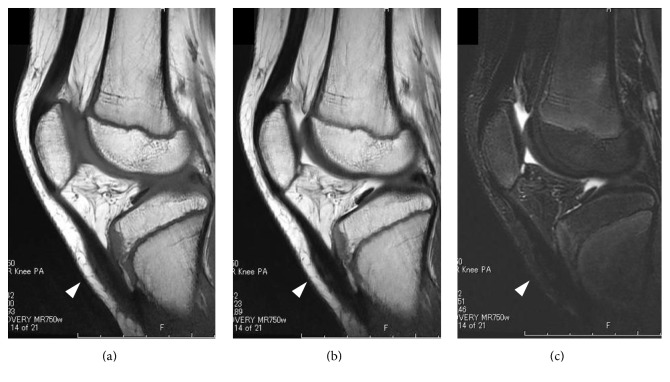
One year after surgery, lateral MRI of the left knee on (a) a T1-weighted image, (b) a T2-weighted image, and (c) a STIR image. The white arrowhead indicates the tendinous continuity of the patellar tendon.
